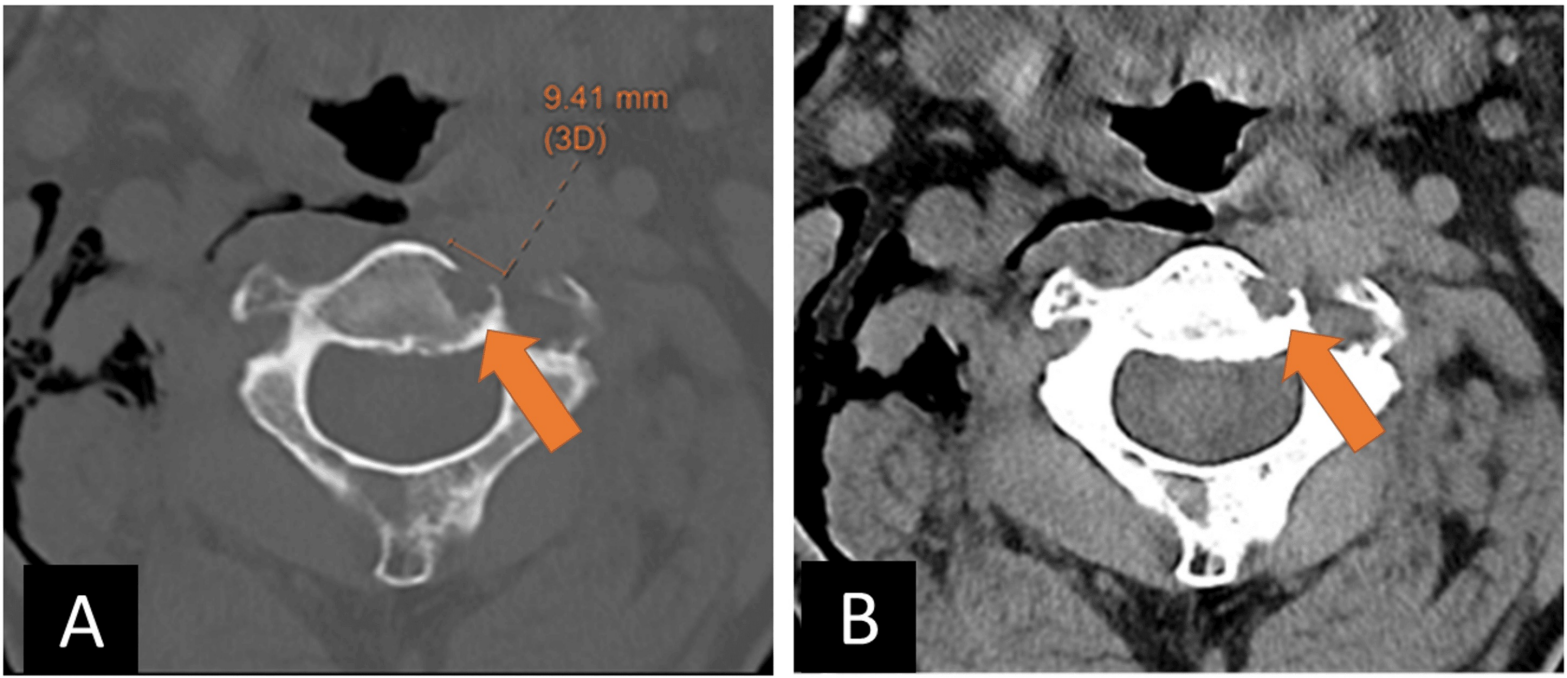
An incidental lytic lesion of the ventral C2 body with dehiscence of the anterior cortex, without appreciable soft tissue extension. Note subcutaneous emphysema of the right neck from trauma-related pneumothorax. A) axial bone window CT; B) axial soft tissue window CT.
